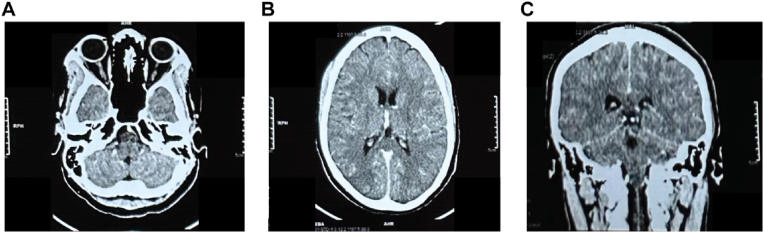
Contrasted head CT from day 3 showing A) no retinal hemorrhage; B) No large territory ischemia, intracerebral hemorrhage, mass effect, or hydrocephalus; C) herniation as an alternative explanation of acute vision loss.
